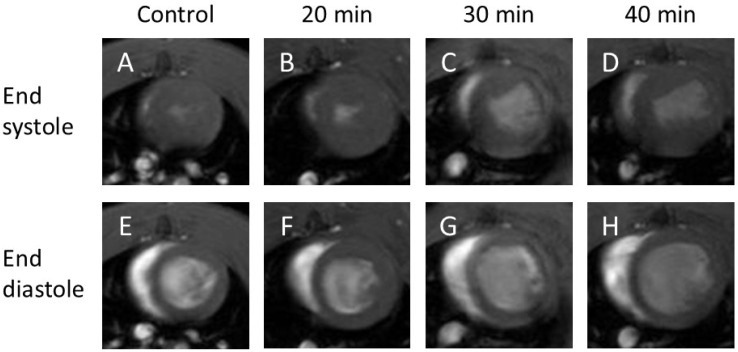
Cine-MR images. Cine images of end-systole (A–D) and end-diastole (E–H). At end-systole, infarction at 30 min (C) and 40 min (D) showed no contraction compared with the control (A) and 20 min infarction (B). At end-diastole, infarction at 30 min (G) and 40 min (H) showed increased volume compared with the control (E) and 20 min infarction (F).
